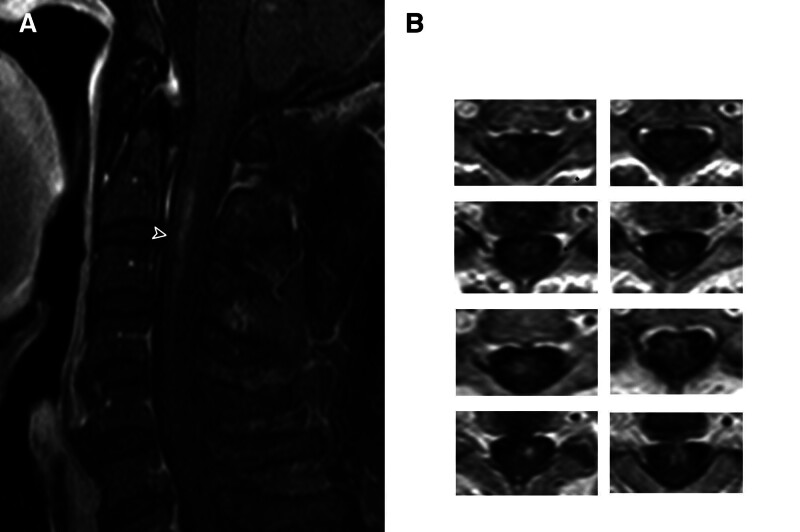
Spinal cord infarction features on contrast-enhanced MRI. (A) Sagittal T1-weighted post-contrast image: linear gadolinium enhancement along the anterior spinal artery distribution (arrows) spanning C2 to C4. (B) Axial T2-weighted image: bilateral symmetric hyperintensity in the anterior horns (“owl’s eye” sign).
